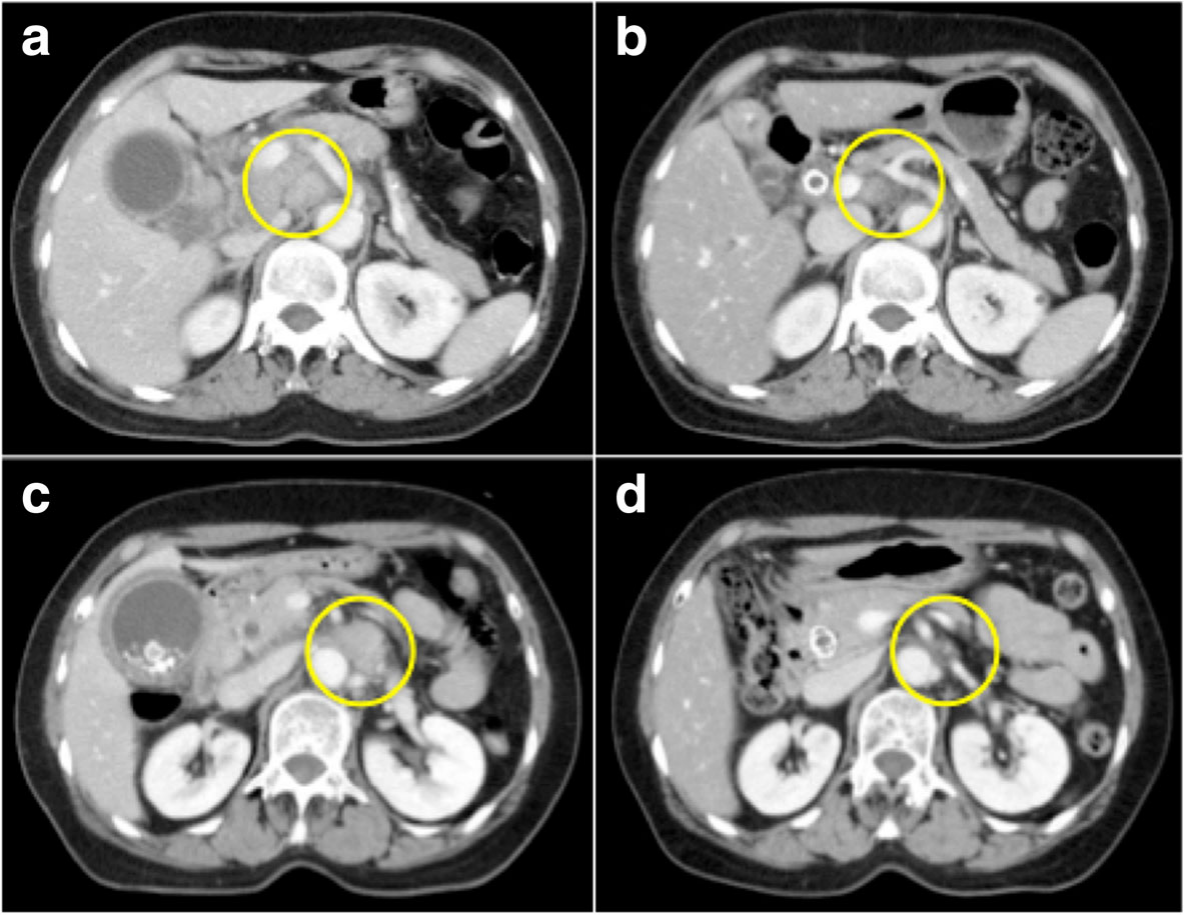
Abdominal CT images scan showing swollen regional lymph nodes before chemotherapy (a, b), and after 32 courses of chemotherapy (c, d). Maximal diameter measurements of the regional lymph nodes were decreased by more than 50%: from 20 mm before chemotherapy (a) to 5 mm after chemotherapy (c) for celiac lymph node #9 and from 20 mm before chemotherapy (b) to 8 mm after chemotherapy (d) for para-aortic lymph node #16a2 inter
